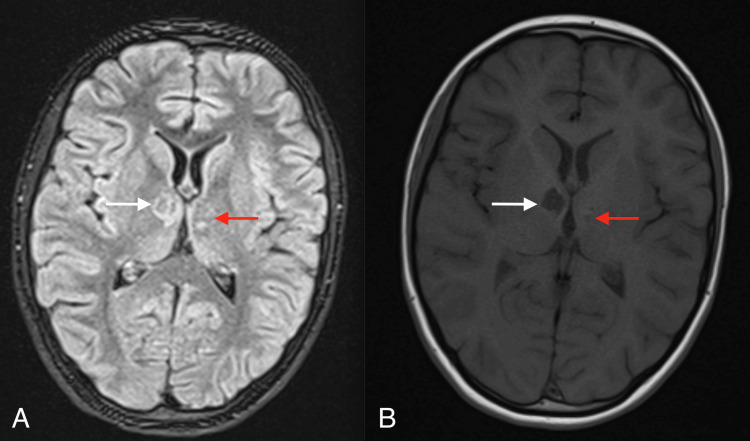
Week 18 from symptom onset. Follow-up brain MRI performed in the Infectious Diseases Department shows an area of malacia in the basal ganglia and thalamus (white arrow) and small foci of porencephaly in the left basal ganglia (red arrow) on T2 FLAIR (A) and post-contrast T1-weighted imaging (B).
